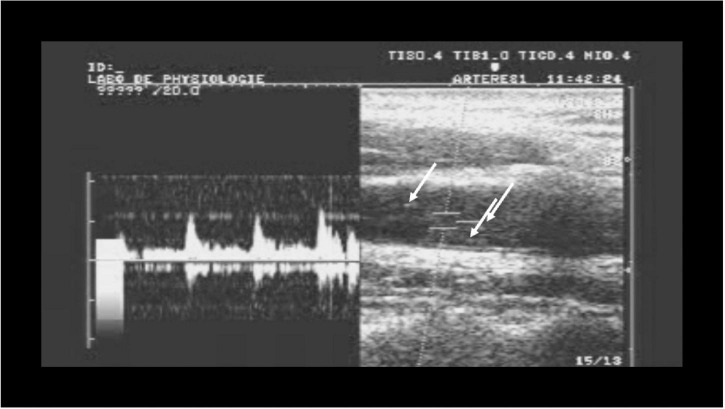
Simultaneous Pulsed Wave Doppler-mode and B-mode ultrasound over the carotid artery, showing bubbles (arrows) passing through the imaging field producing the “gurgling” sound heard over the carotid artery with Doppler.
